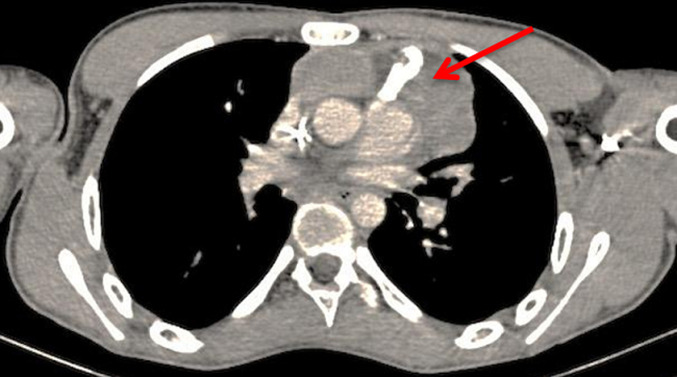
chest computed tomography scan after chemotherapy demonstrating a partial response
